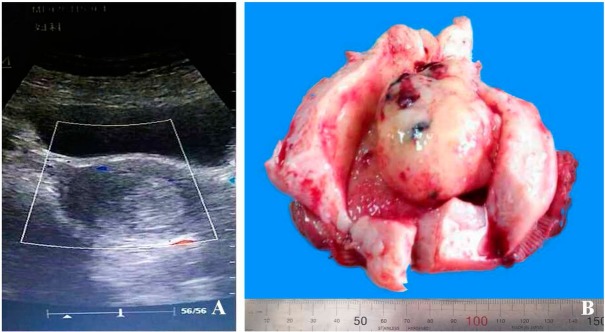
Imaging and gross feature of the case. A) Ultrasound images showing that the uterine cavity was occupied by an oval shaped mass. B) The gross appearance of the brown polypoid tumor located in the uterine cavity and originated from the endometrium.
